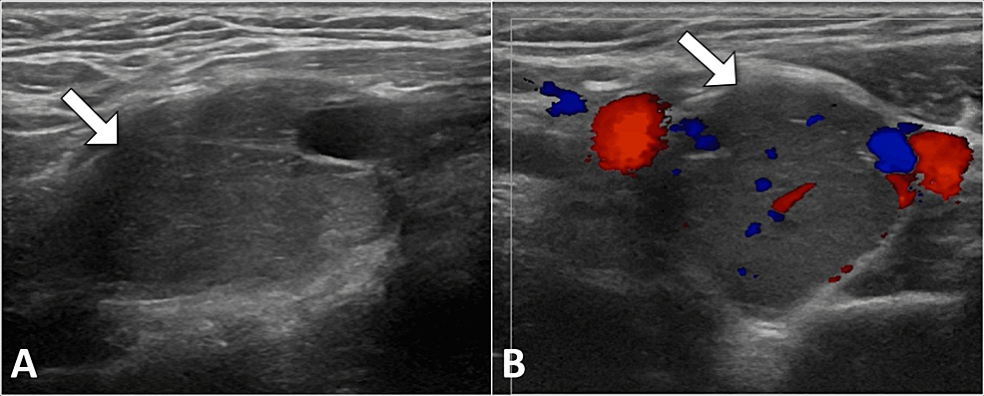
Longitudinal grayscale (A) and transverse color Doppler views of the neck displaying a solid mass (arrow) causing displacement of the internal and external carotid arteries without invasion.
